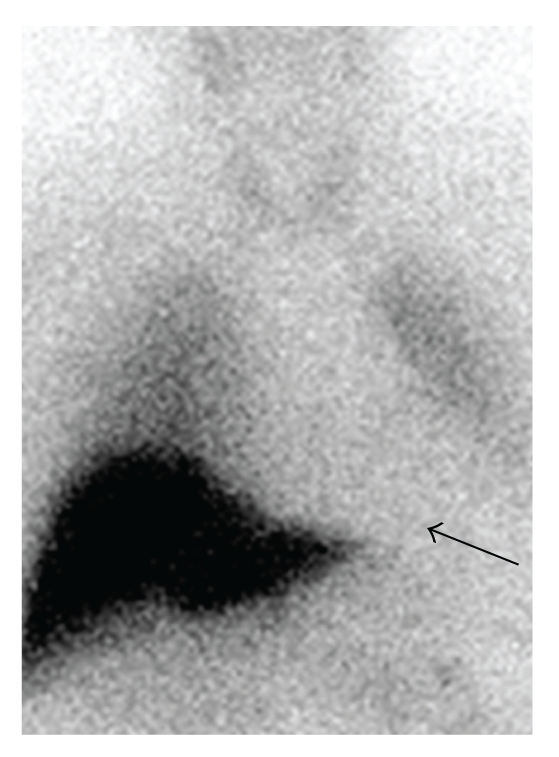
MIBG scintigraphy in akinetic rigid PD. MIBG scintigraphy in a 65-year old male patient with akinetic rigid type of PD, Hoehn and Yahr stage I. There is hardly any myocardial MIBG uptake (black arrow).
